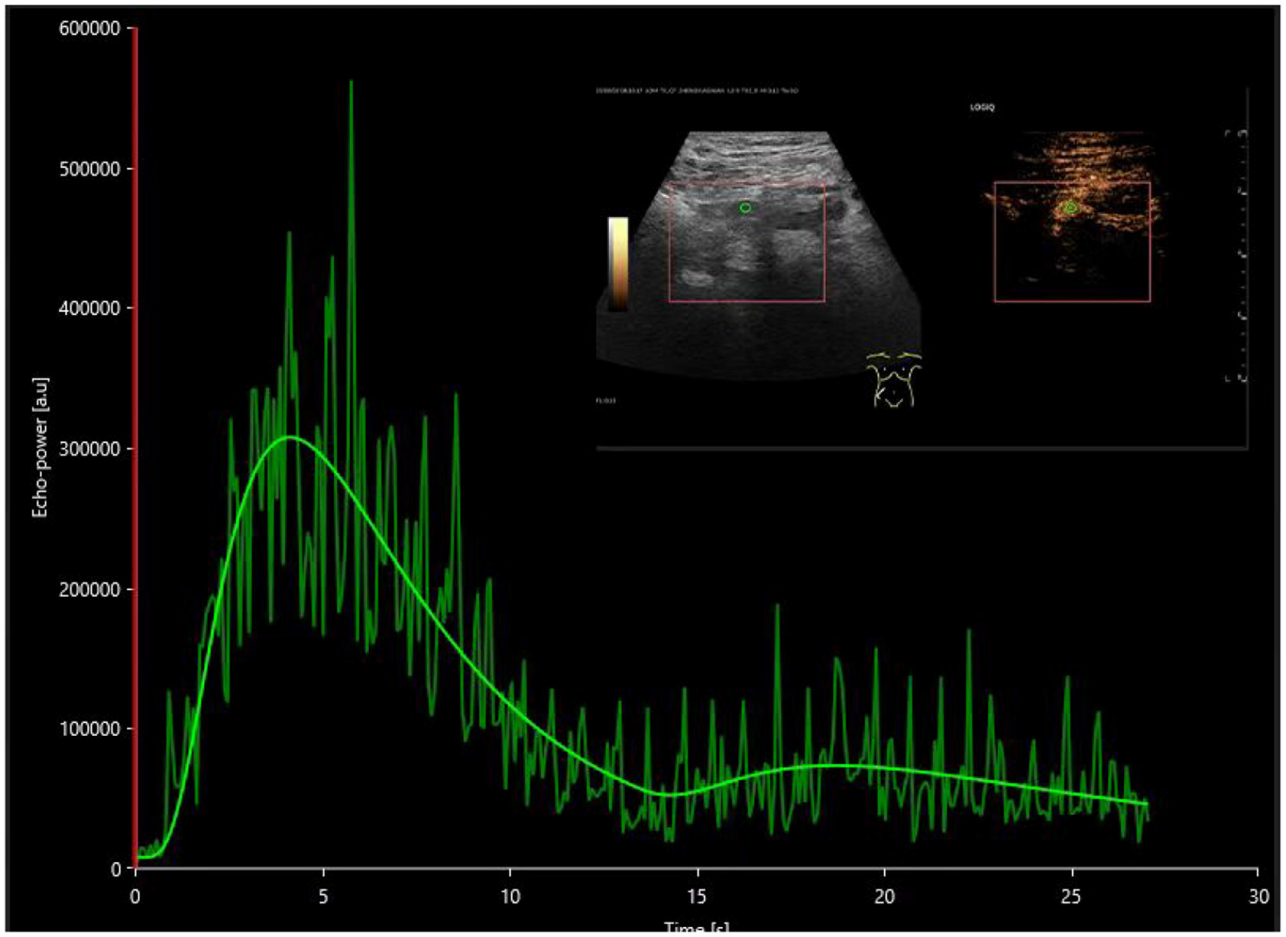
The time-intensity curve of the main renal artery on contrast-enhanced ultrasound in the postoperative DGF group.
